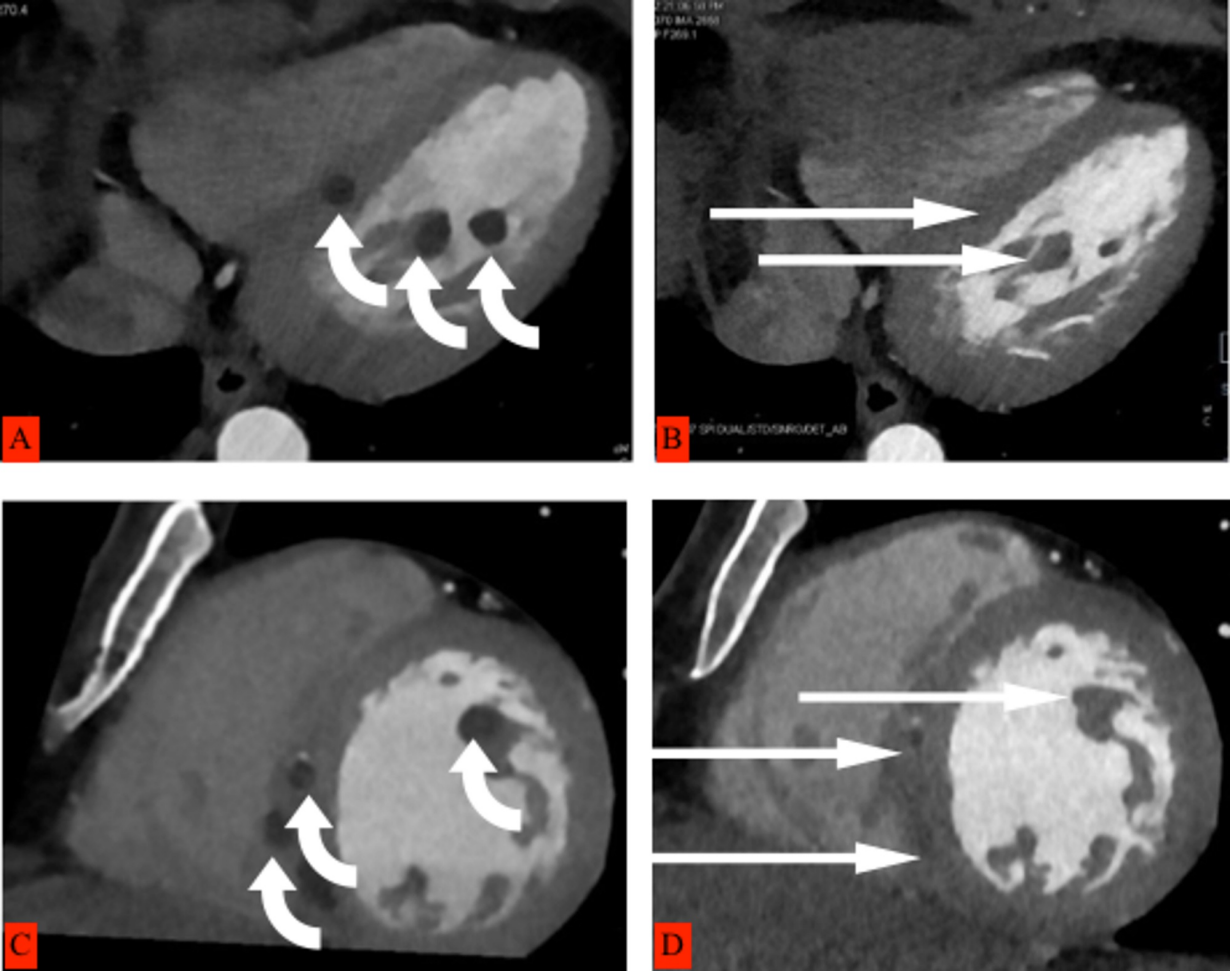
Cardiac CT images on the left side (A, C) showing multiple hypodense cystic lesions pointed with curved arrows at the interventricular septum and papillary muscles before treatment, and on the right side (B, D), significantly smaller and fainter lesions pointed with long arrows six weeks after treatment.
